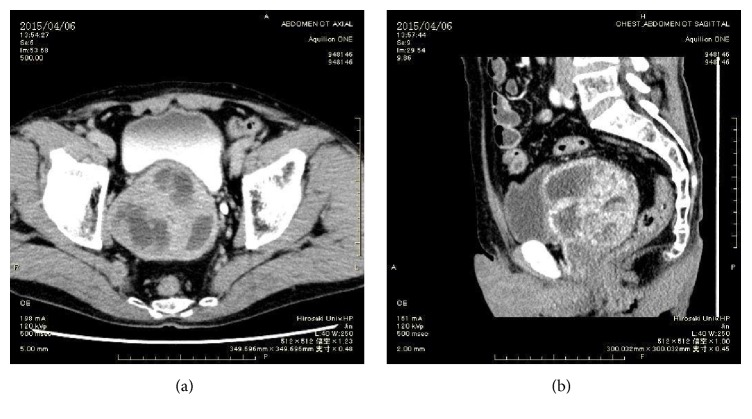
CT scan image of prostatic tumor. (a) Axial image. (b) Sagittal image.
